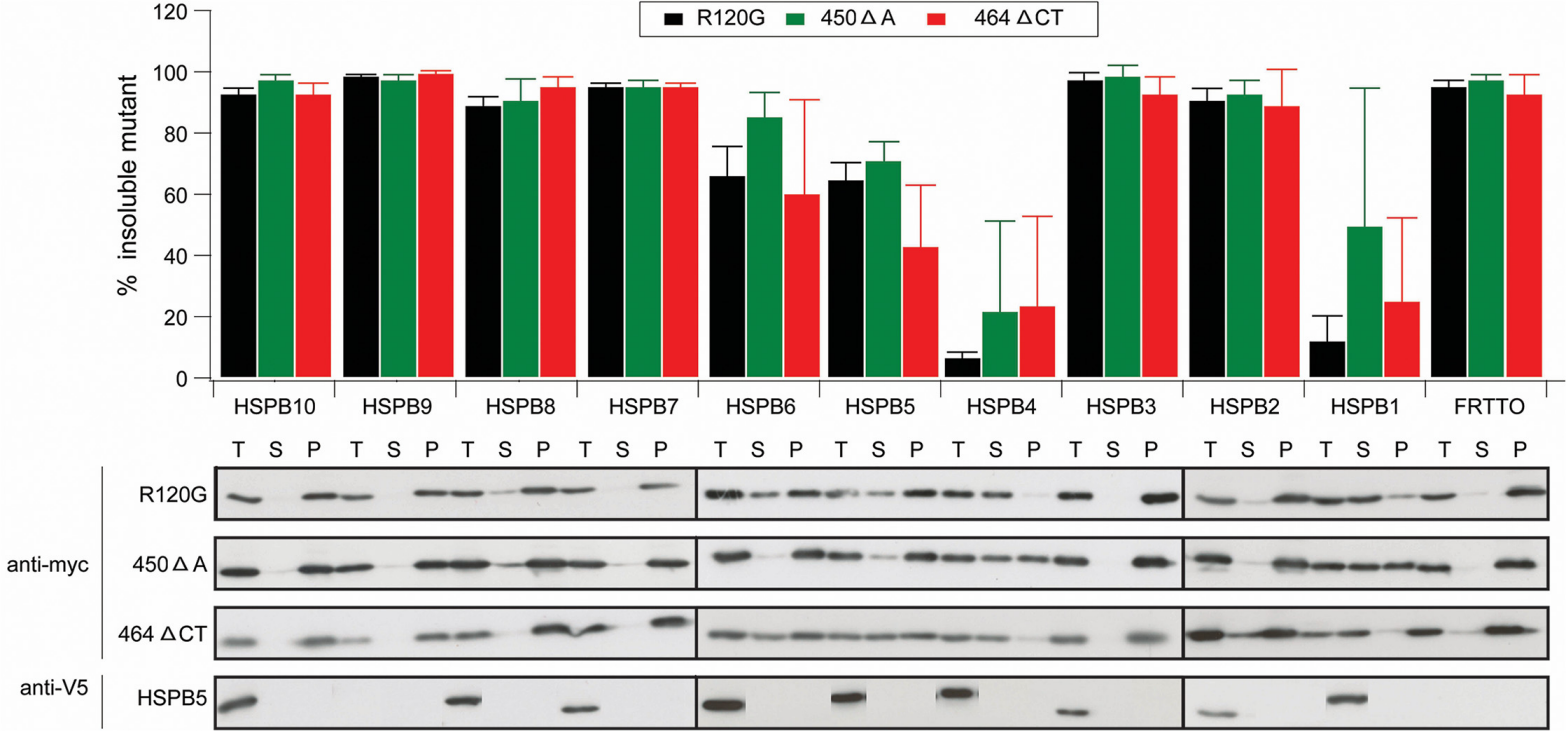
Effect of the 10 human HSPB family members on the aggregation of HSPB5 mutants. HEK293 cells were co-transfected with 0.5 μg of myc- HSPB5 (R120G) or (450 Δ A) or (464 Δ CT) mutants and either FRTTO as a control or V5 tagged HSPBs (HSPB1-10) at 1:3 ratio for 48 h. Cells were lysed with Triton X- 100 lysis buffer, fractionated into S (soluble) and P (pellet) fractions or T (total lysate) and western blotting was performed. The percentage of insoluble HSPB5 mutants in each case was quantified ([p]_density_*100 / ([p] _density_ + [s] _density_)) and is depicted in the chart above the blot. Values represent mean ± SD of 3 independent experiments.
